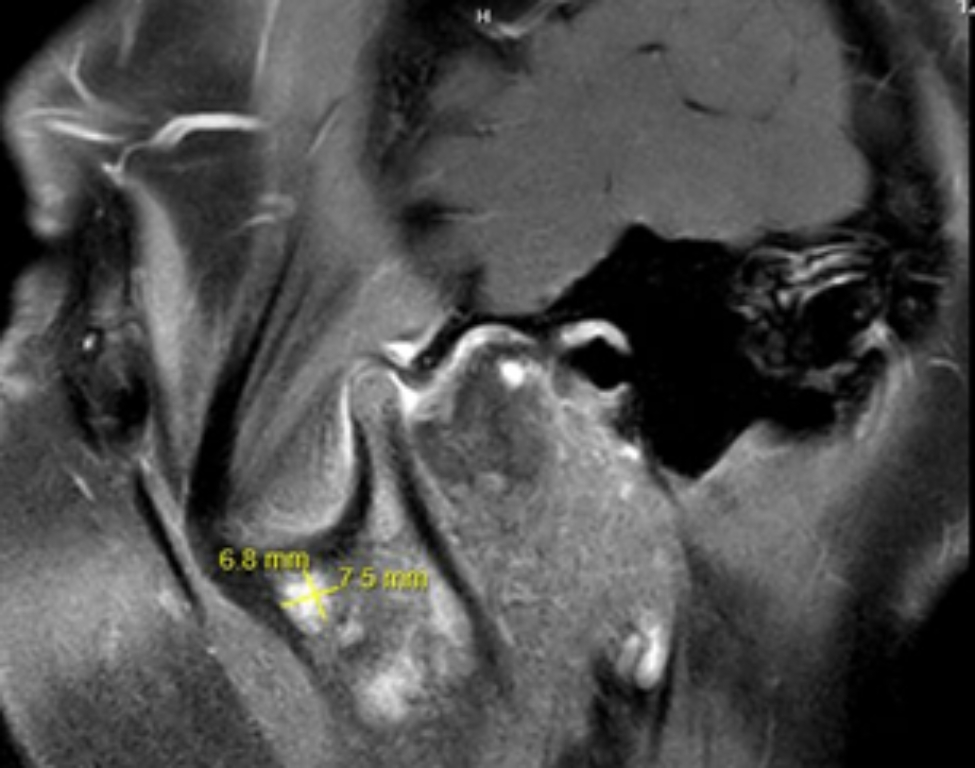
Sagittal oblique fat saturated post open mouth MRI showing T2 hyperintense lesion with enhancement in the subchondral bone extending to the articular cartilage. An additional more ill-defined 6.8 × 7.5 mm lesion is seen anteriorly in the base of the coronoid process
